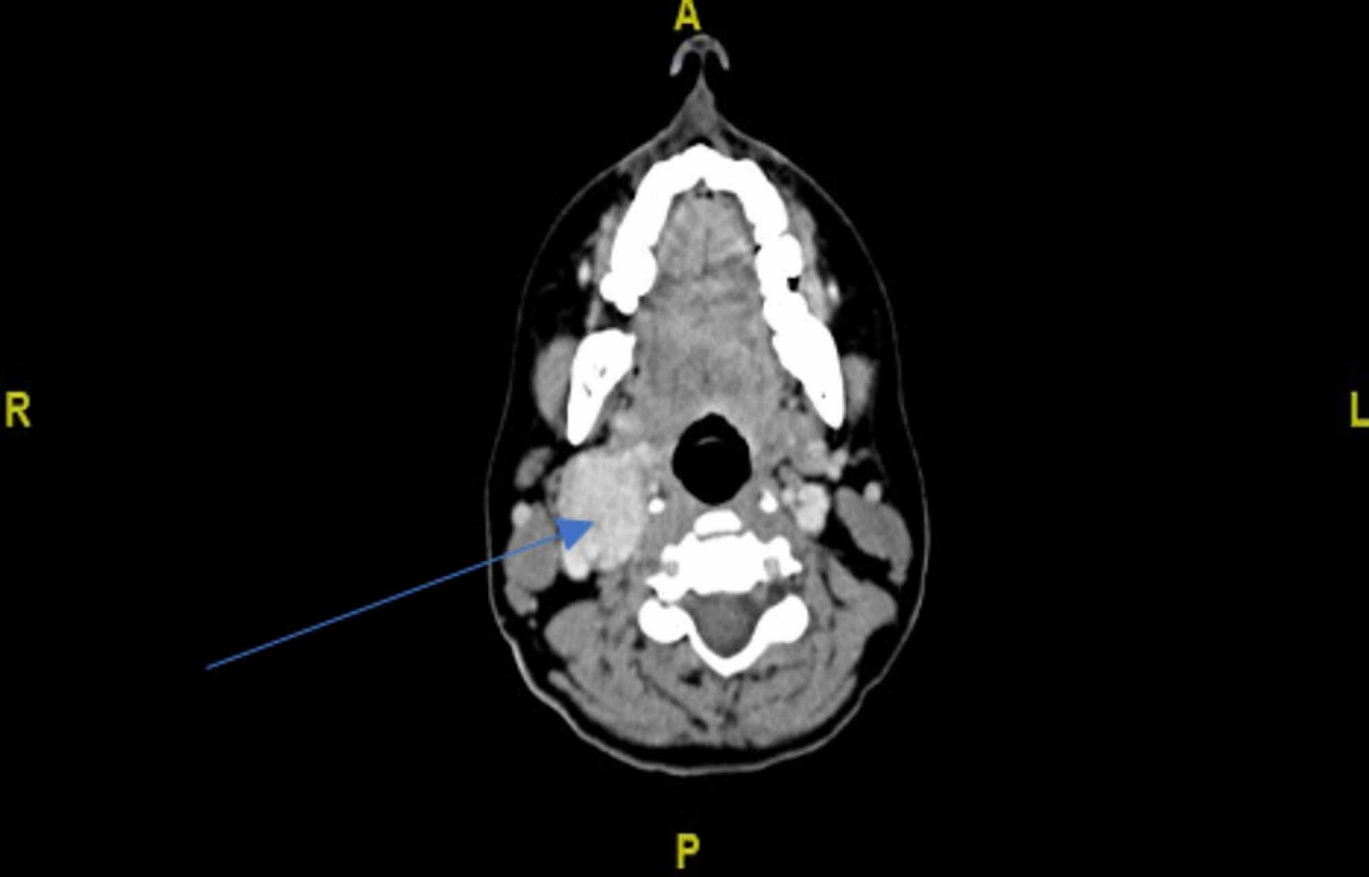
Contrast-enhanced computed tomography demonstrated a focal, rounded soft tissue mass of size 3 X 2.7 X 3.6 cm and located along the bifurcation of the right common carotid artery with splaying of external and internal carotid artery
